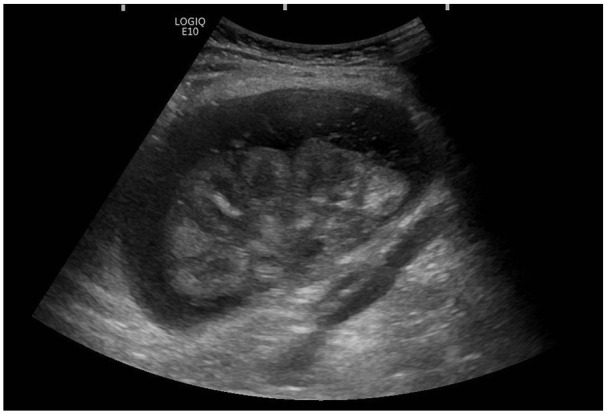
Ultrasound one month later: Shallow 2.5-cm fluid collection containing debris encasing the transplant kidney.
